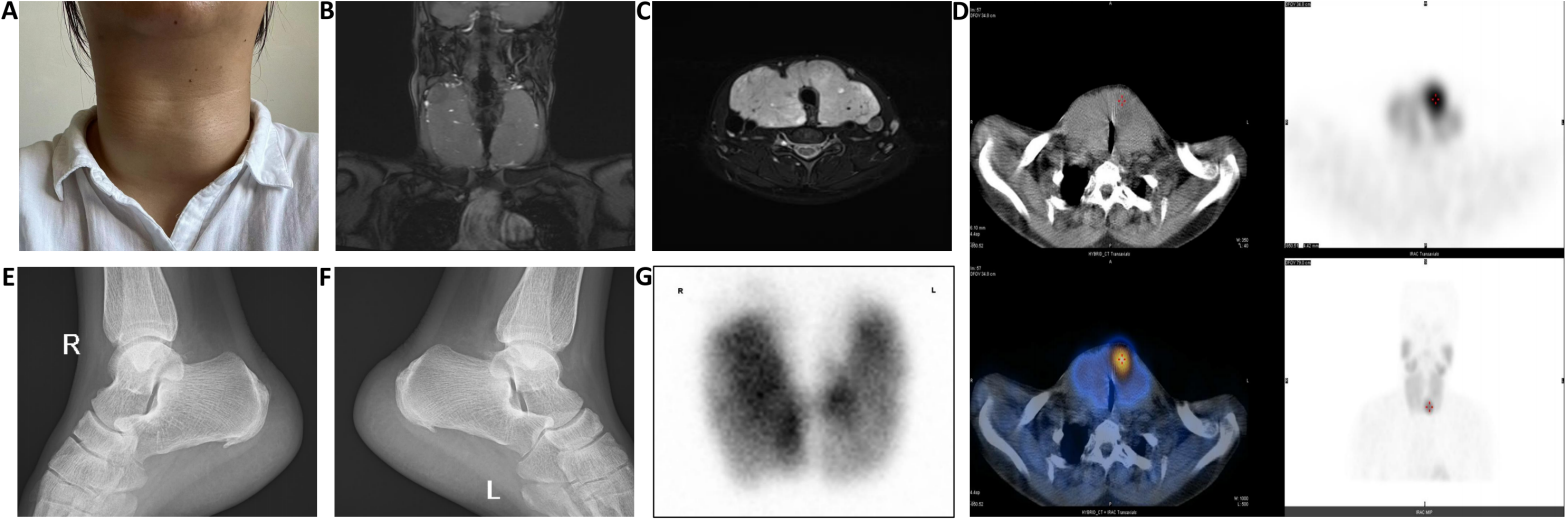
(A) The thyroid volume significantly decreased and became firm after two weeks of preoperative iodine treatment; (B, C) Thyroid MRI shows significant enlargement of the bilateral thyroid lobes and isthmus, with compression and narrowing of the trachea; (D) After intravenous injection of 370 MBq of ^99m^Tc-MIBI, imaging at 15 minutes shows normal positioning of both thyroid lobes with an enlarged shape, an area of abnormally increased tracer uptake is observed near the isthmus at the lower pole of the left lobe, while the remaining lobes show uneven tracer distribution, at 120 minutes post-injection, the area of increased tracer uptake near the isthmus at the lower pole of the left lobe persists, with significantly reduced tracer distribution in the rest of both lobes, no other areas of abnormal tracer concentration are observed within the field of view; (E, F) X-ray findings show bone hyperplasia of the right and left calcaneus; (G) After intravenous injection of 185 MBq of ^99m^TcO_4_
